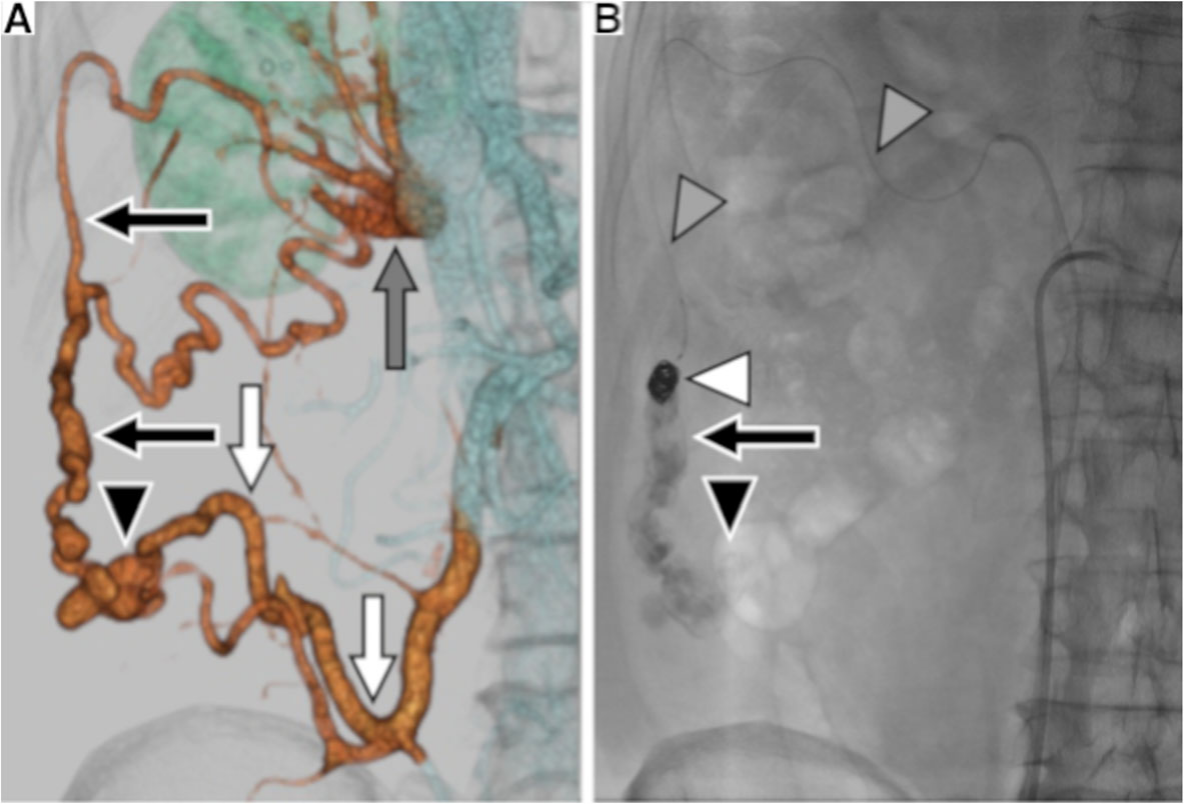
Overviews of anatomy and treatment. a Three-dimensional volume rendering image (VR image) reconstructed from contrast medium-enhanced CT. The image shows the ascending colonic varix (black arrowhead), the main draining vein (black arrows), and the ileocolic vein (white arrows) as a feeder vein. The gray arrow shows the right renal vein. b Overview X-ray image. After injection of the sclerosing agent, microcoils (white arrowhead) are inserted via the microballoon catheter (gray arrowheads). When the balloon is deflated, contrast medium is retained in the colonic varix (black arrowhead) and the draining vein (black arrow)
